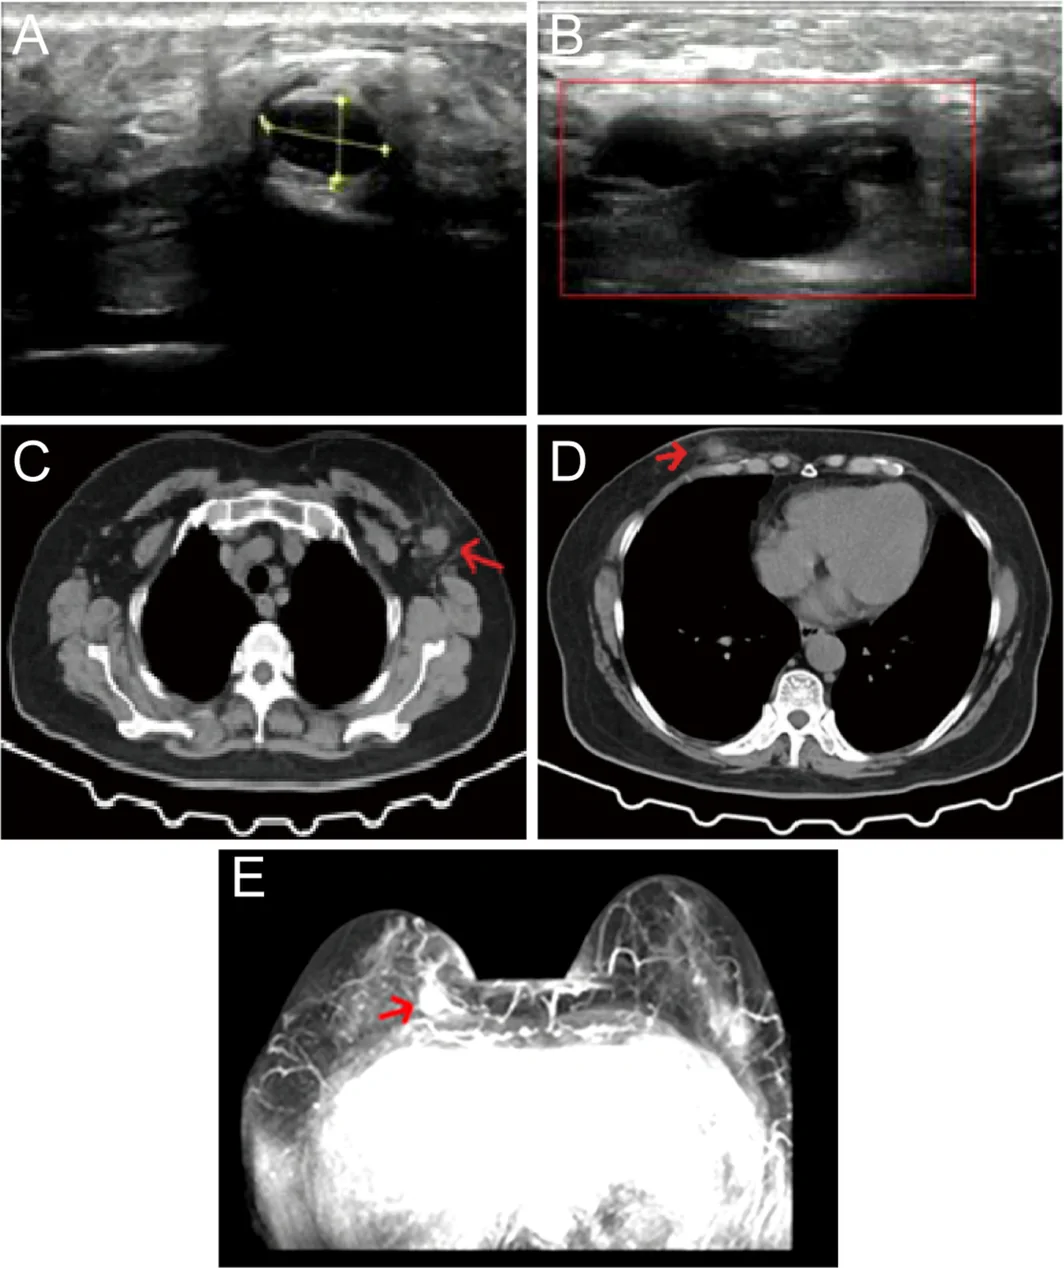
Multimodal imaging of the right breast lesion and left axillary lymph node involvement. (A) Breast ultrasound showing a mixed‐echogenic nodule at the 5 o'clock position of the right breast. (B) Ultrasound of the left axilla showing an enlarged lymph node suspicious for metastasis. (C) Contrast‐enhanced CT of the chest showing enlarged lymph nodes in the left axilla. (D) Contrast‐enhanced CT of the chest showing a nodule in the inner lower quadrant of the right breast. (E) Contrast‐enhanced breast MRI showing an enhancing lesion in the right breast.
